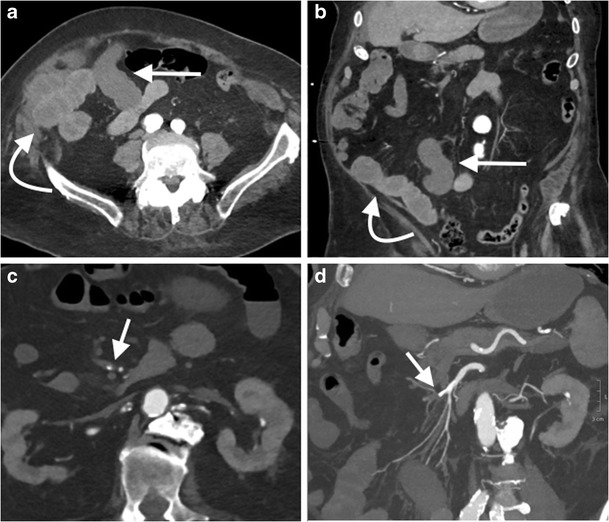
An 85-year-old woman developed severe abdominal pain two days post percutaneous aortic valve replacement and was referred for a CT mesenteric angiogram. Axial (a) and coronal (b) images from an arterial phase CT mesenteric angiogram demonstrate hypoenhancement of distal ileal loops (*straight arrow*) compared with adjacent proximal ileal and jejunal loops with normal mural enhancement (*curved arrow*). Axial (c) and coronal oblique images from the same study demonstrate focal occlusion of the mid-superior mesenteric artery with calcified plaque (*arrow*). Coronal oblique maximum intensity projection (MIP) reformat (d) is useful in demonstrating the point of SMA obstruction (*arrow*). Exploratory laparoscopy revealed extensive small bowel ischaemia, and the patient unfortunately expired
